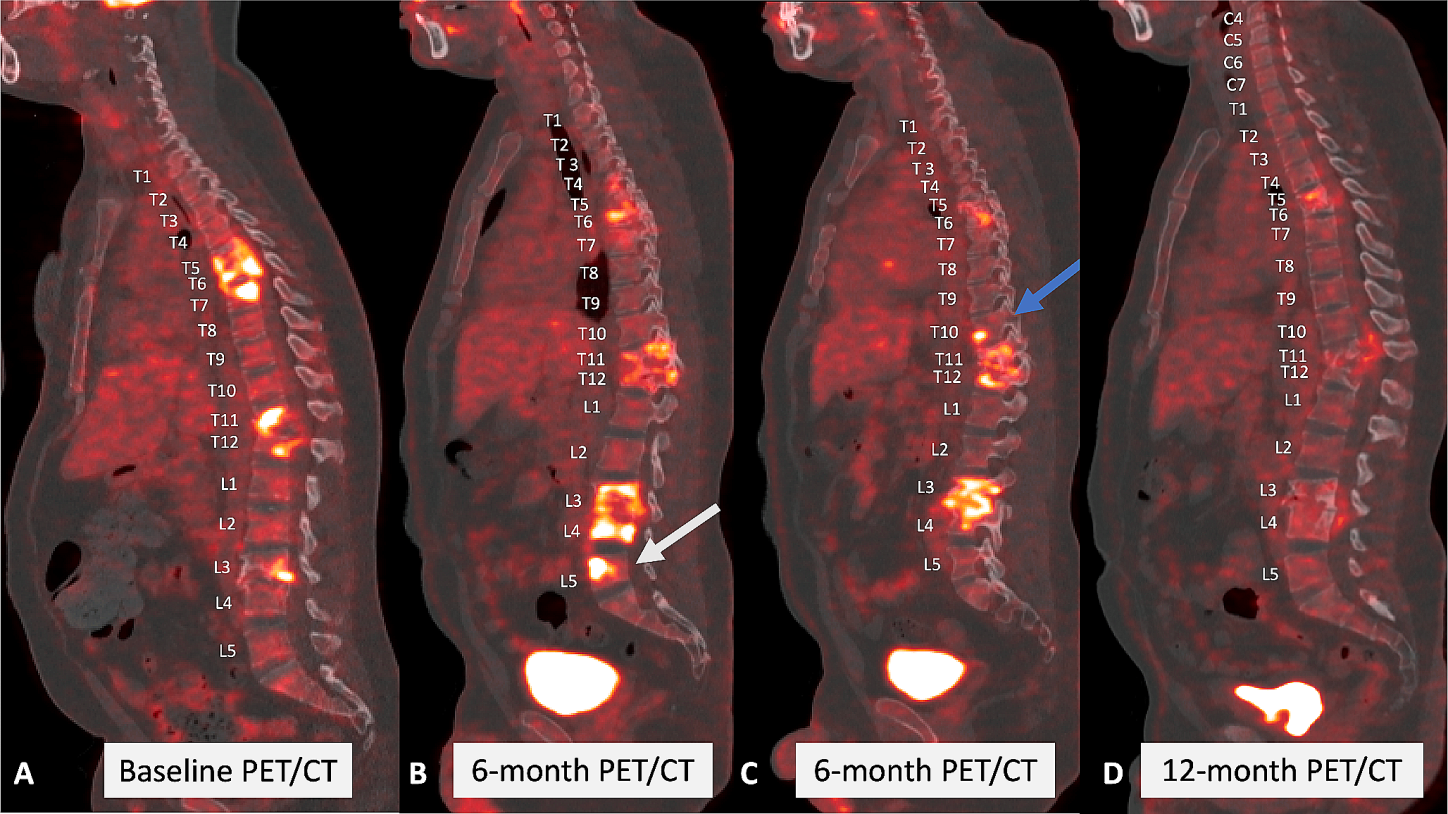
Comparison of initial, 6-months and 12-months follow-up spinal PET/CT (bone window) A: Sagittal view of initial PET/CT. B: Sagittal view of 6-months follow-up; white arrow: new L5 lesion. C: Sagittal view of 6-months follow-up; blue arrow: new Th10 lesion. D: Sagittal view of 12-months follow-up
